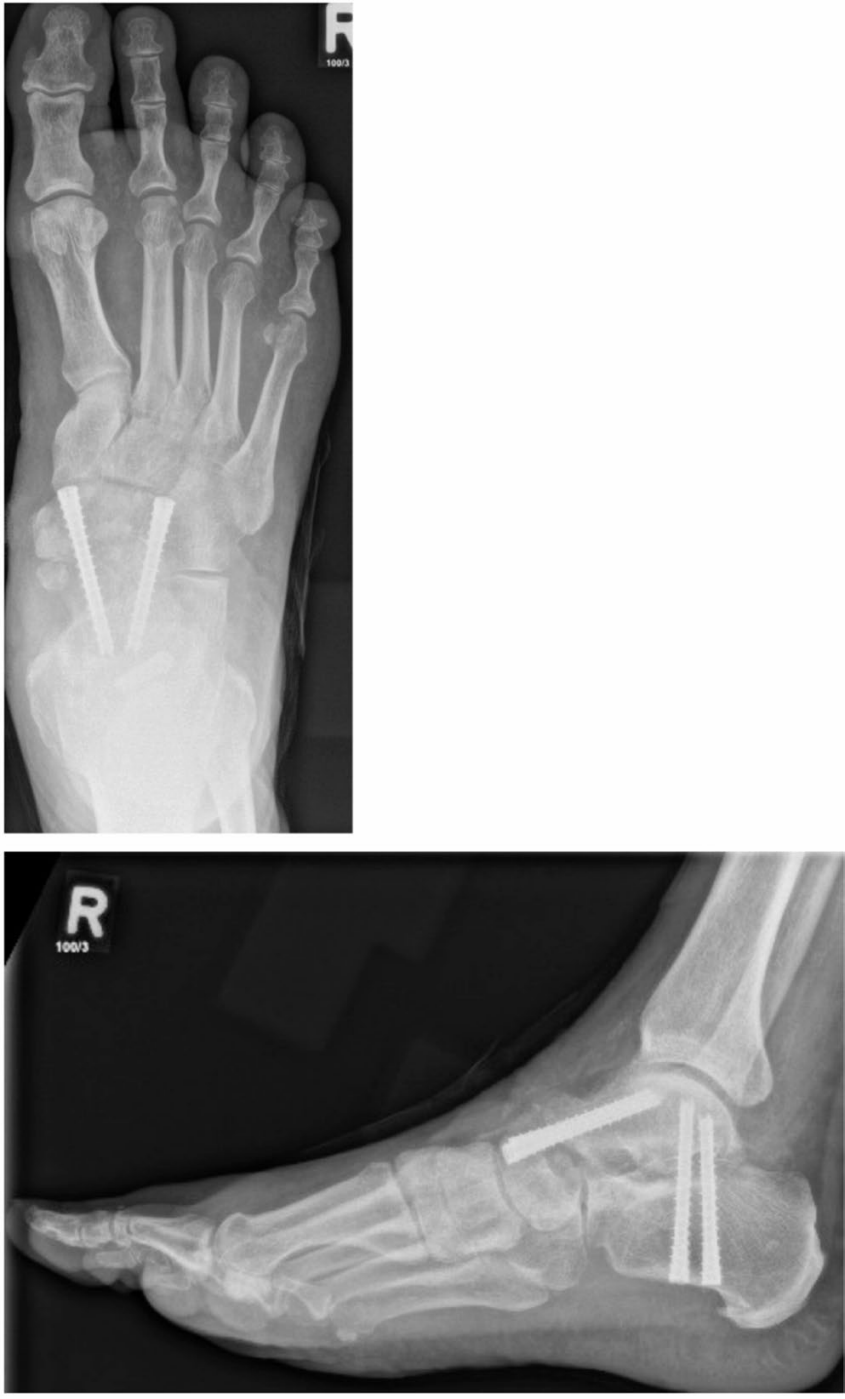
Postoperative radiographs after MIS TN arthrodesis: dorsoplantar projection (left) and lateral projection (right). In this case, a concomitant MIS subtalar arthrodesis was additionally performed
